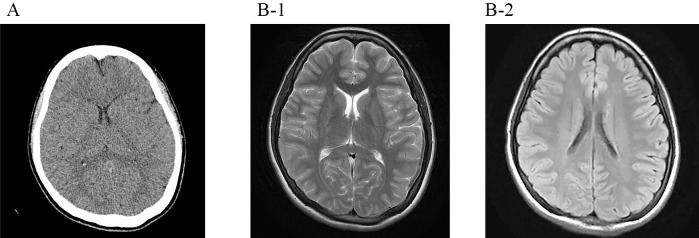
Head imaging findings. A: Plain head CT scan (axial section). B: Plain head MRI axial section (B-1: T2 weighted imaging B-2: fluid-attenuated inversion recovery pattern). No obvious abnormalities were observed on head imaging on the day of symptom onset.
